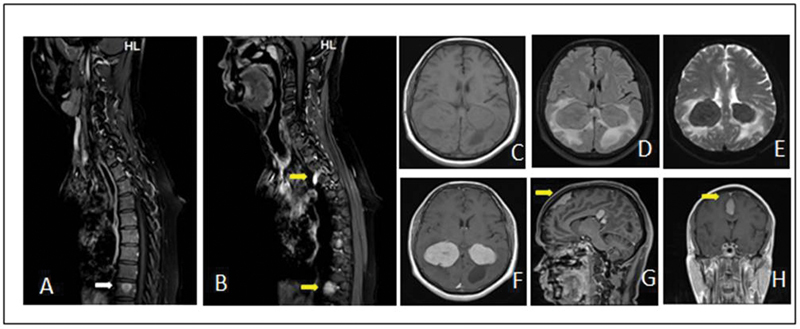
Magnetic resonance imaging (MRI) spine and brain plain and contrast study: (
